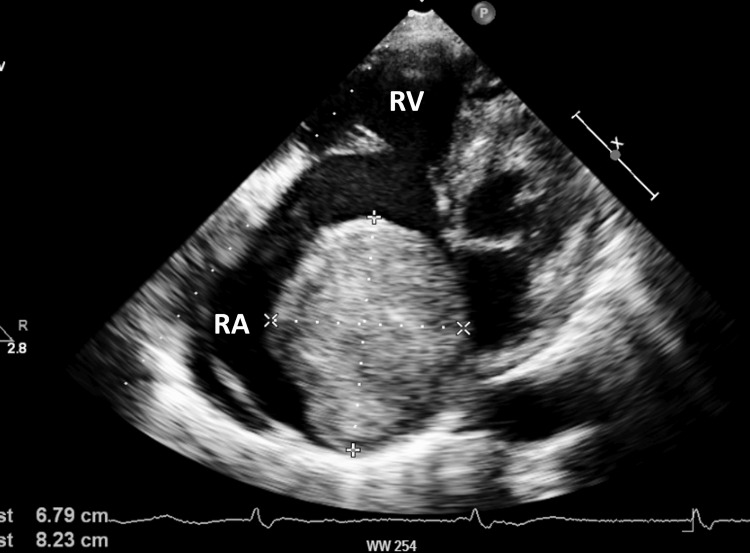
Preoperative echocardiography Preoperative echocardiography shows a large atrial myxoma (82 mm × 68 mm) in the right atrium. RA: Right atrium; RV: Right ventricle.
